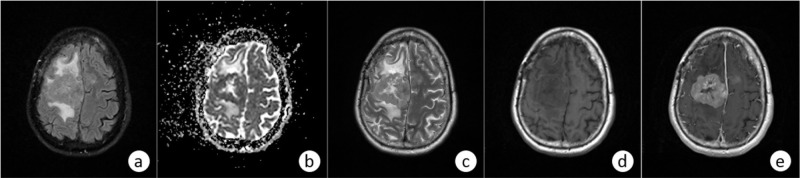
The study used images acquired for each enrolled patient using five different MRI pulse sequences: (a) FLAIR; (b) ADC; (c) T2-W; (d) and (e) DCE pre- and postcontrast, respectively ADC: apparent diffusion coefficient; DCE: dynamic contrast-enhanced; FLAIR: fluid-attenuated inversion recovery; MRI: magnetic resonance imaging; T2-W: T2-weighted
